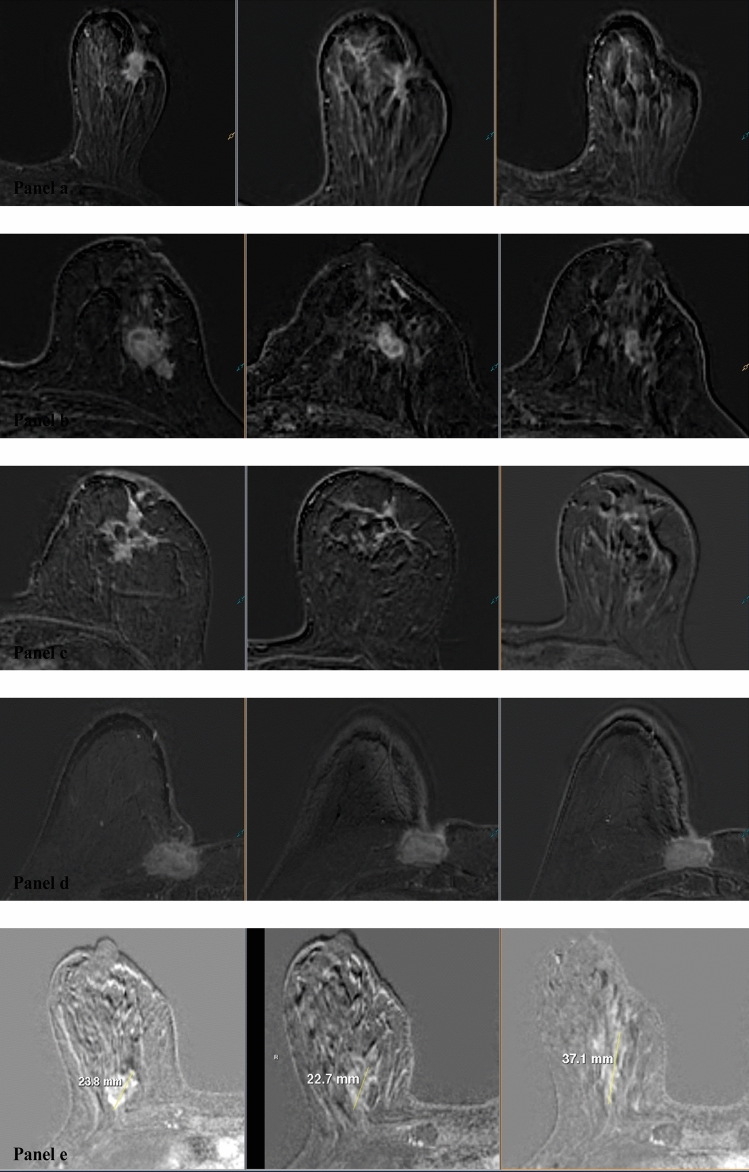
MRI morphological response patterns of ER + /HER2- LABC at three different time points (from left to right): baseline, between regimens (after 2 months of treatment), and presurgery (after 4 months of treatment); a a tumor that shrinks concentrically after 2 months with NET, while at the end of therapy, the patient achieved a complete imaging response; b a tumor that shows concentric shrinkage without surrounding lesion following 2 and 4 months with NET; c a tumor that shows fragmentation pattern throughout the intended therapy; d MRI shows a concentric pattern of tumor regression after 2 months, but reveals a stable disease after 4 months with NET, and e progressive disease. *ER* estrogen receptor; *HER2* human epidermal growth factor receptor 2; *LABC* locally advanced breast cancer, *MRI* magnetic resonance imaging, *NET* neoadjuvant endocrine therapy
